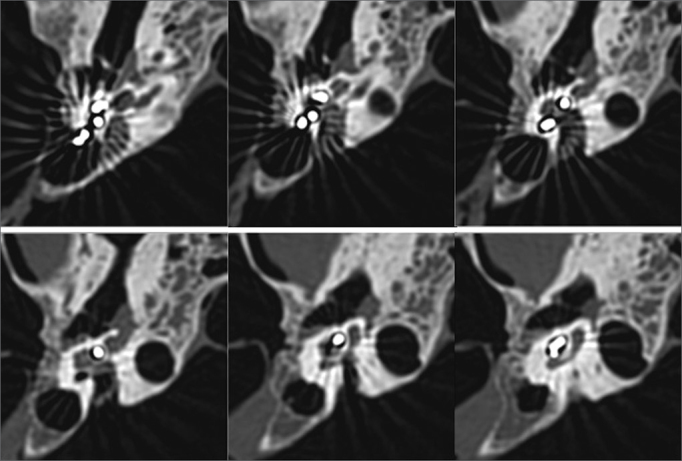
Right temporal bone high-resolution CT scan. Axial view, bone window, showing the placement of the array from the basal to the apical turn of the cochlea.
